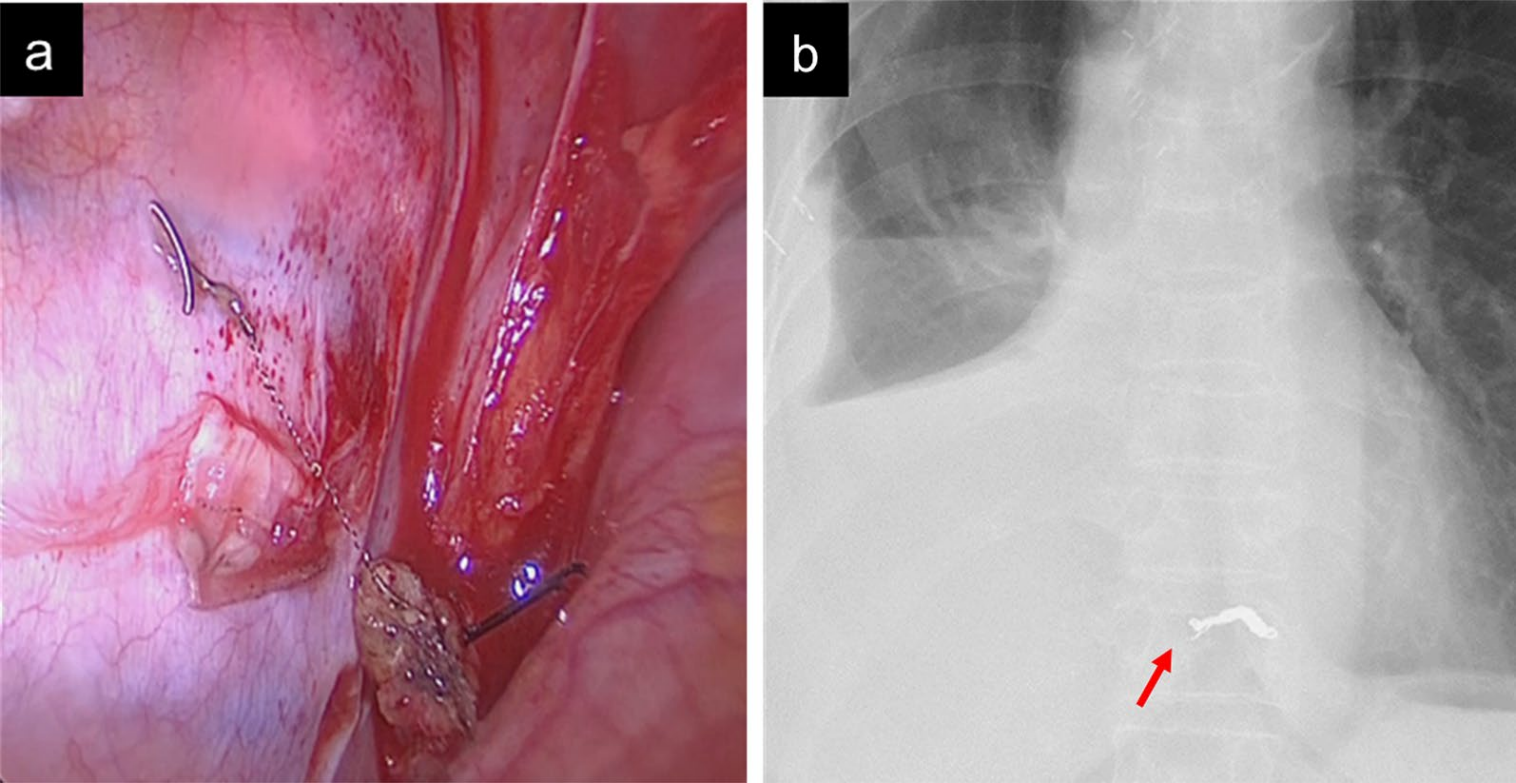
a Intraoperative finding. After the arterial wall was dissected, the coil stump was exposed. b Postoperative chest radiography showing that the coil stump was cut (arrow) and that a part of the coil remained
